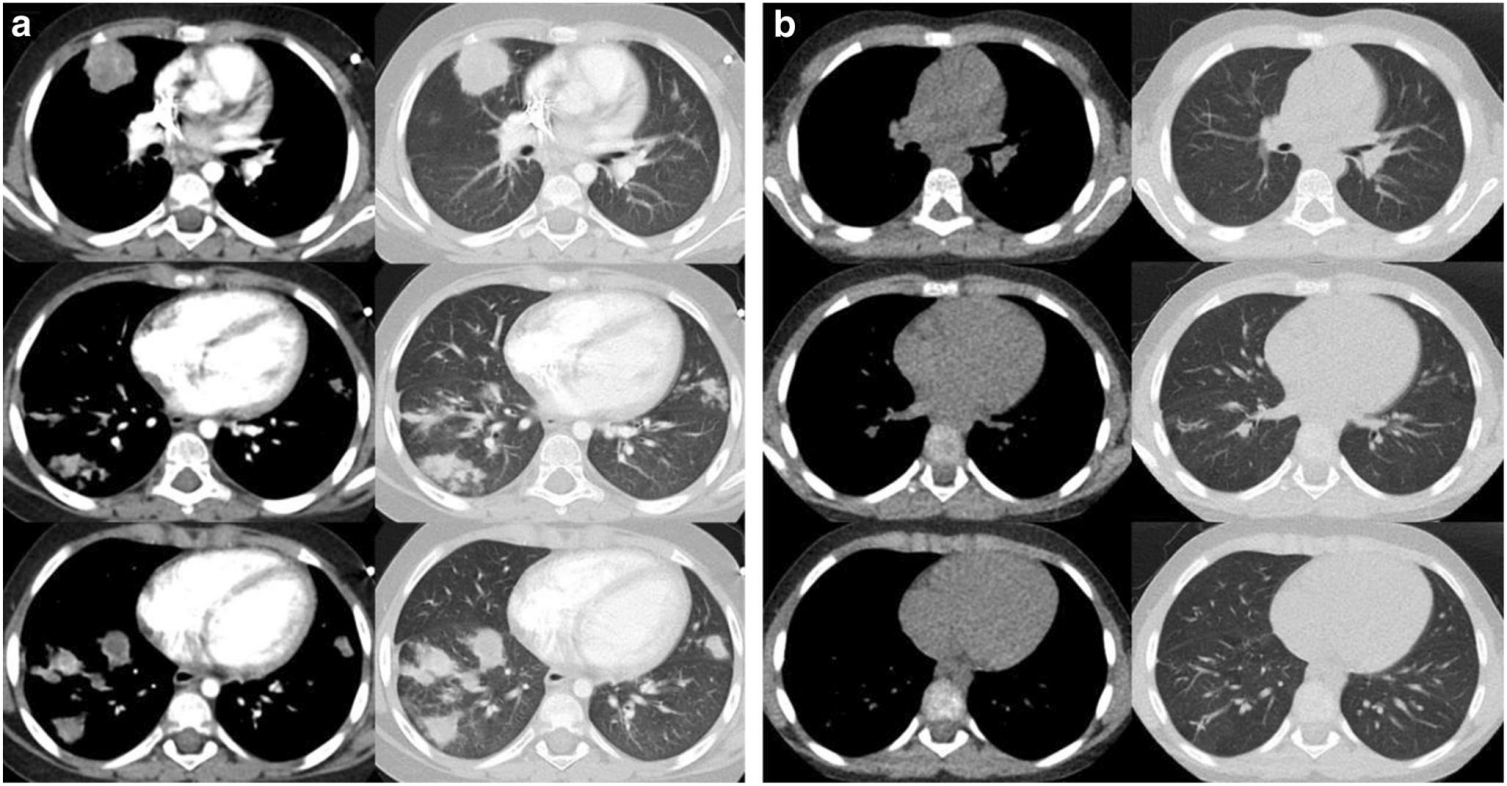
Initial (a) CT scan of the chest and at last follow-up (b). Soft tissue windowing images are displayed in the left and corresponding lung windowing images in the right rows. In the initial series (a), multiple, partly enhancing nodules in both lungs with a maximum diameter of 2.1 cm, predominantly in the lung periphery were detected. Furthermore, two consolidations with a maximum extent of 4.7 cm were present in the lingula and the right lower lobe. At last follow-up (b), only a few subtle residual changes in the lingula and the right lower lobe were seen
